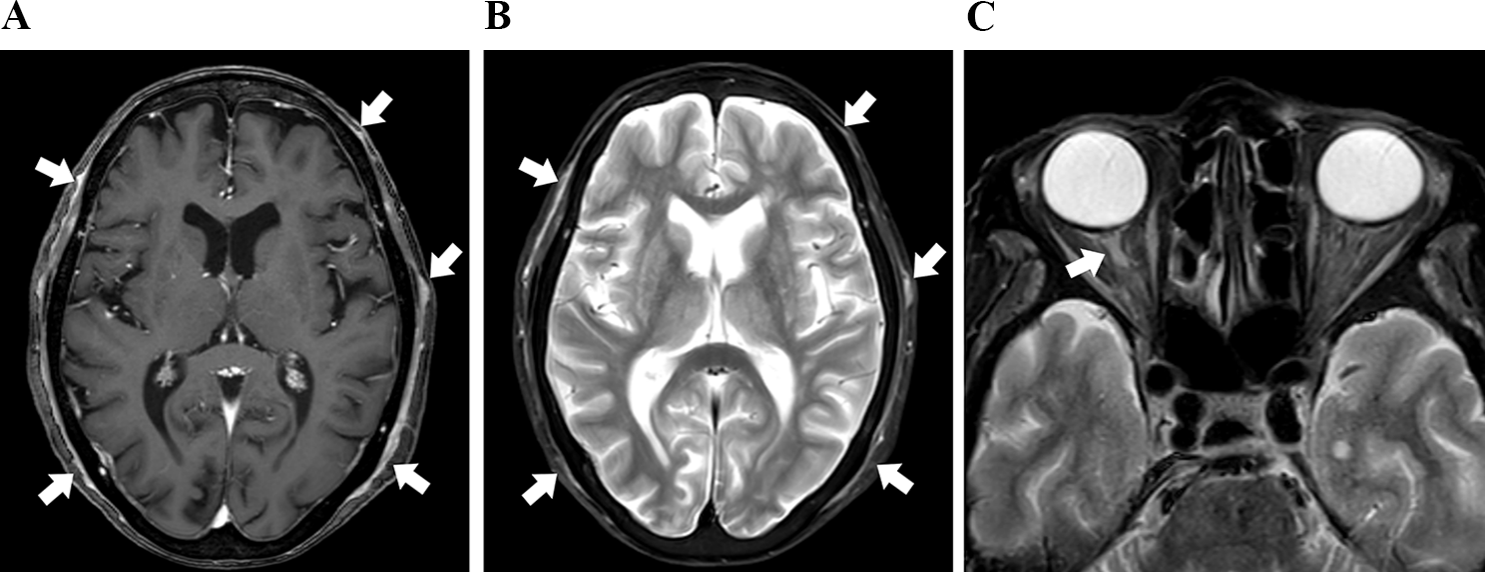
Case 10 (giant cell arteritis). (a), Contrast-enhanced T1-weighted (CE-T1W) image shows multiple distant arcuate enhancement areas of extracranial soft tissues. (b), STIR image shows multiple distant high signal intensity areas of extracranial soft tissues. (c), STIR shows high signal intensity of the right retrobulbar optic nerve
